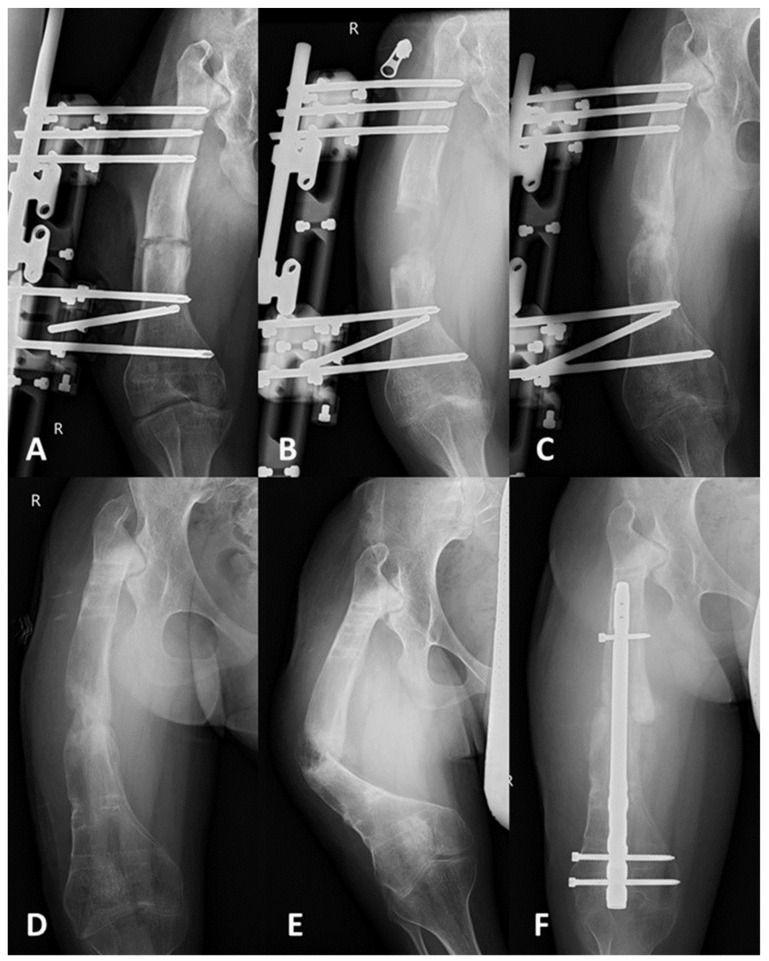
AP radiographs of a 15-year-old patient with CFD and 45 mm aim of lengthening, qualified for femur lengthening with external fixator MRS. (A) Postoperative AP radiograph. (B) AP radiograph after the end of distraction (before the regenerate consolidation). (C) Partial regenerate consolidation. (D) Progress in consolidation, radiograph after the frame removal. (E) Regenerate fracture was observed 27 days after the frame removal and recommended partial weight bearing in a cast. (F) Fracture stabilization with retrograde solid nail—2 mm distraction was subsequently lost to this treatment.
